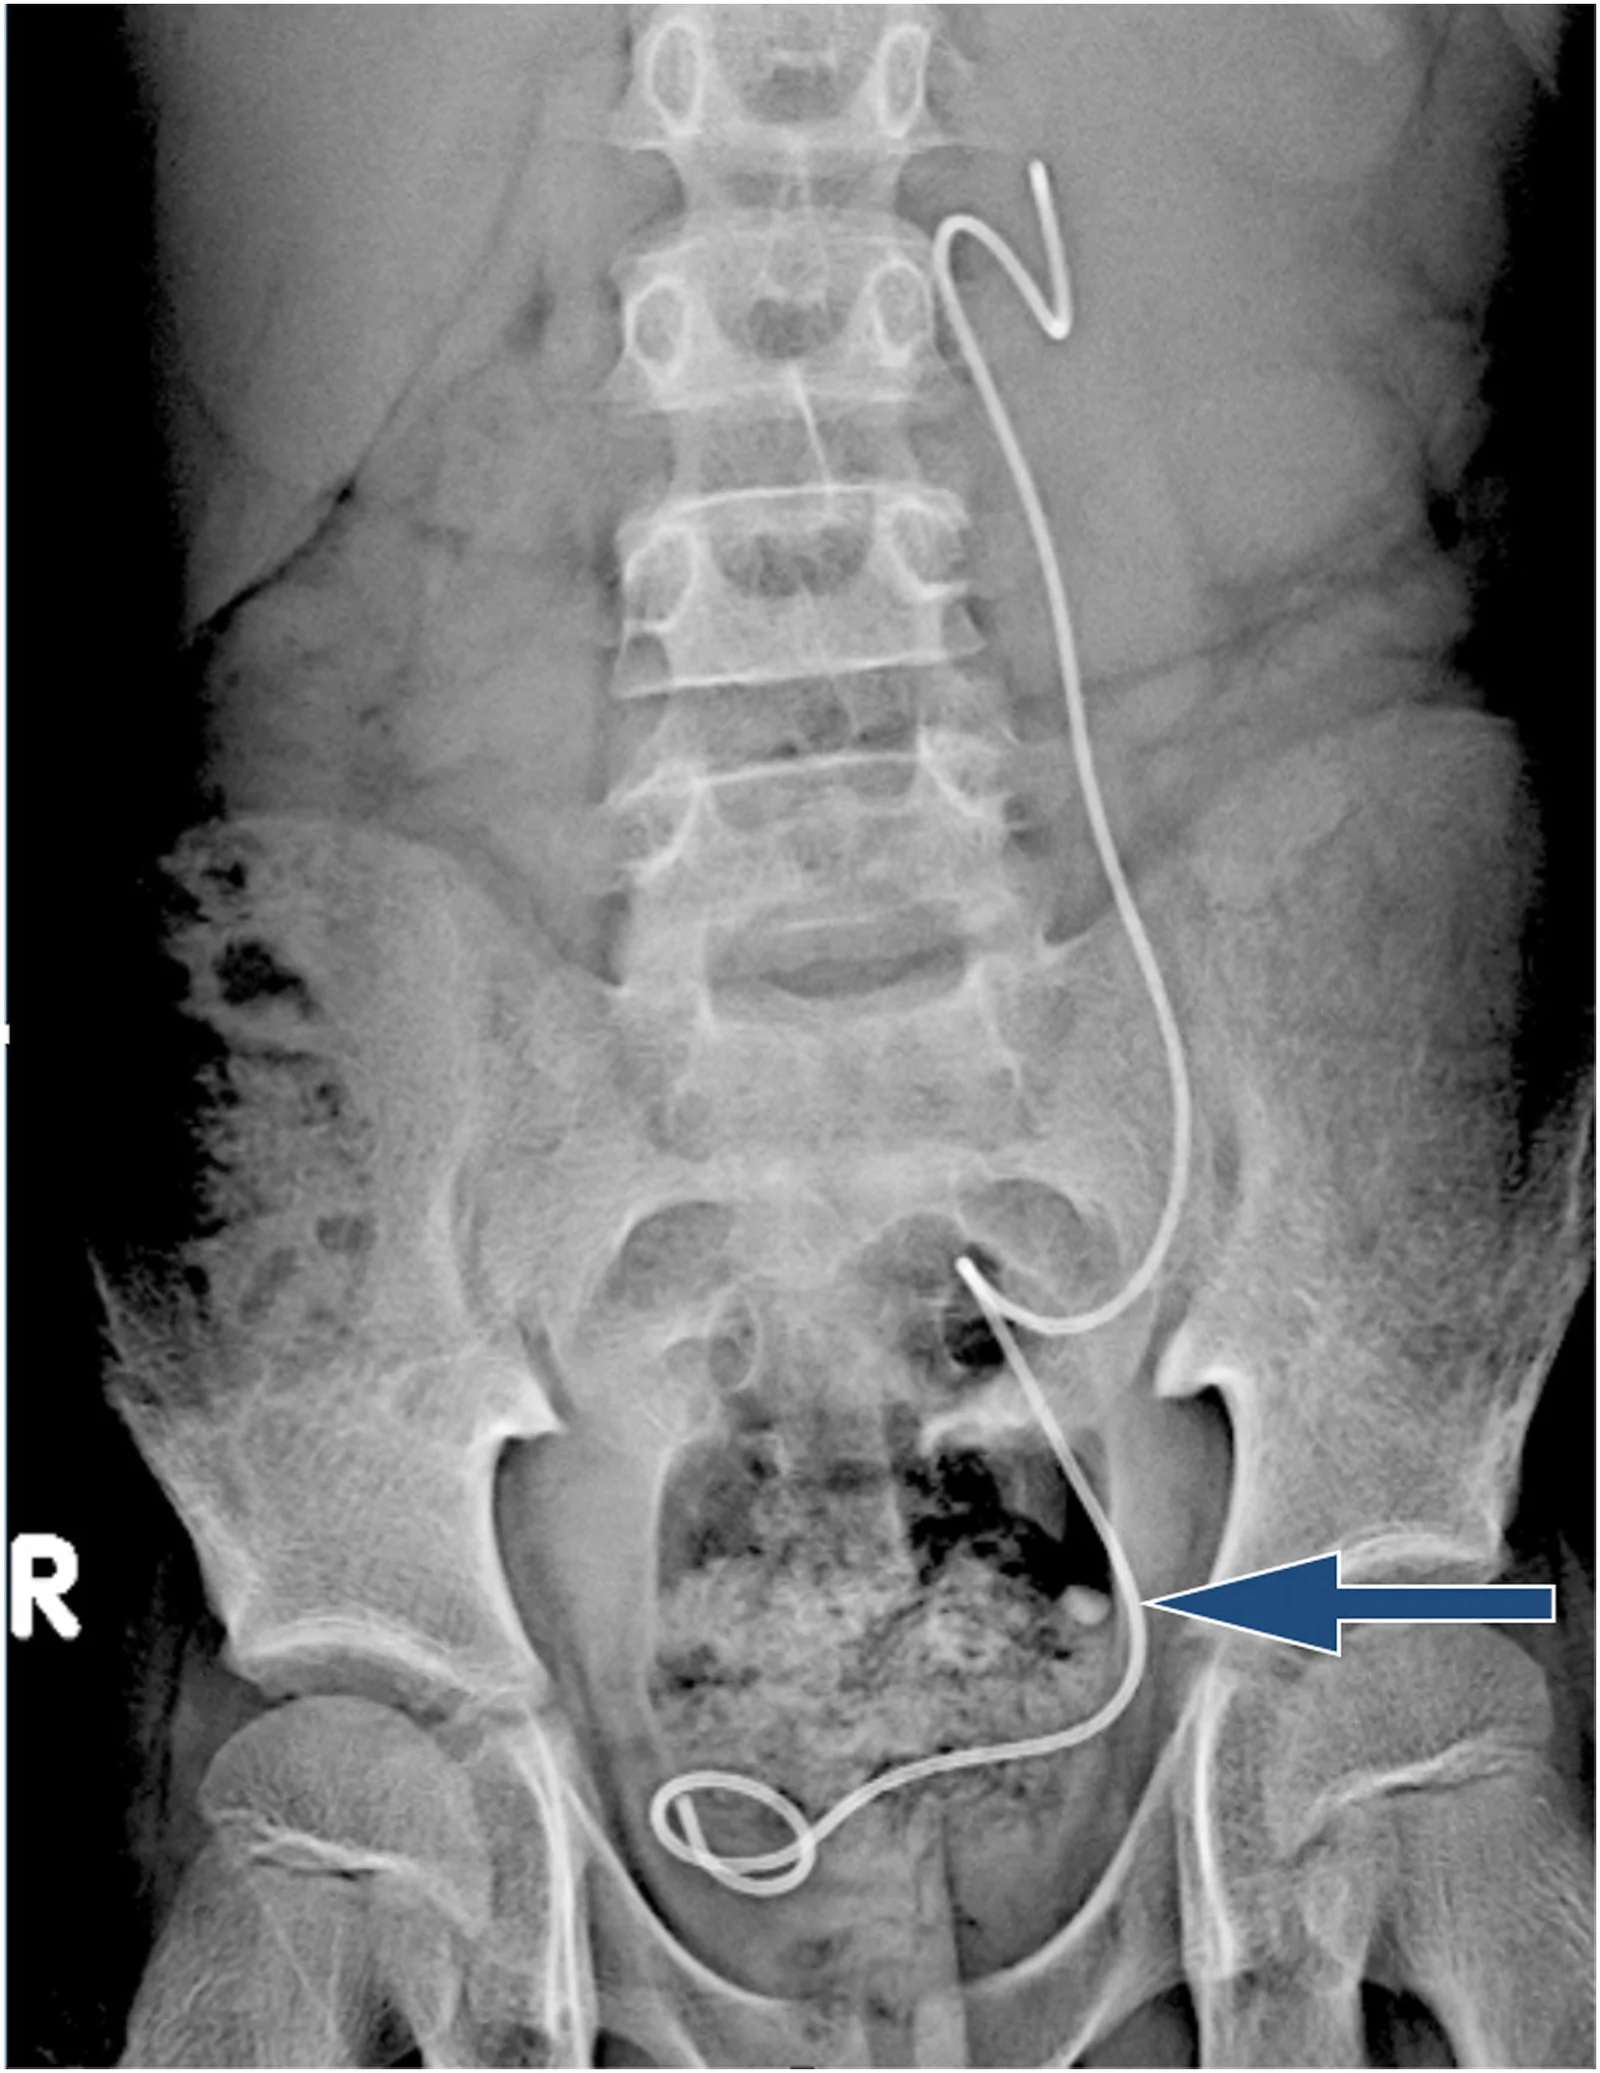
Plain KUB radiograph at six weeks showing the calculus (BLUE arrow) located in the left lower ureter once again, with the double-J stent in situ.
